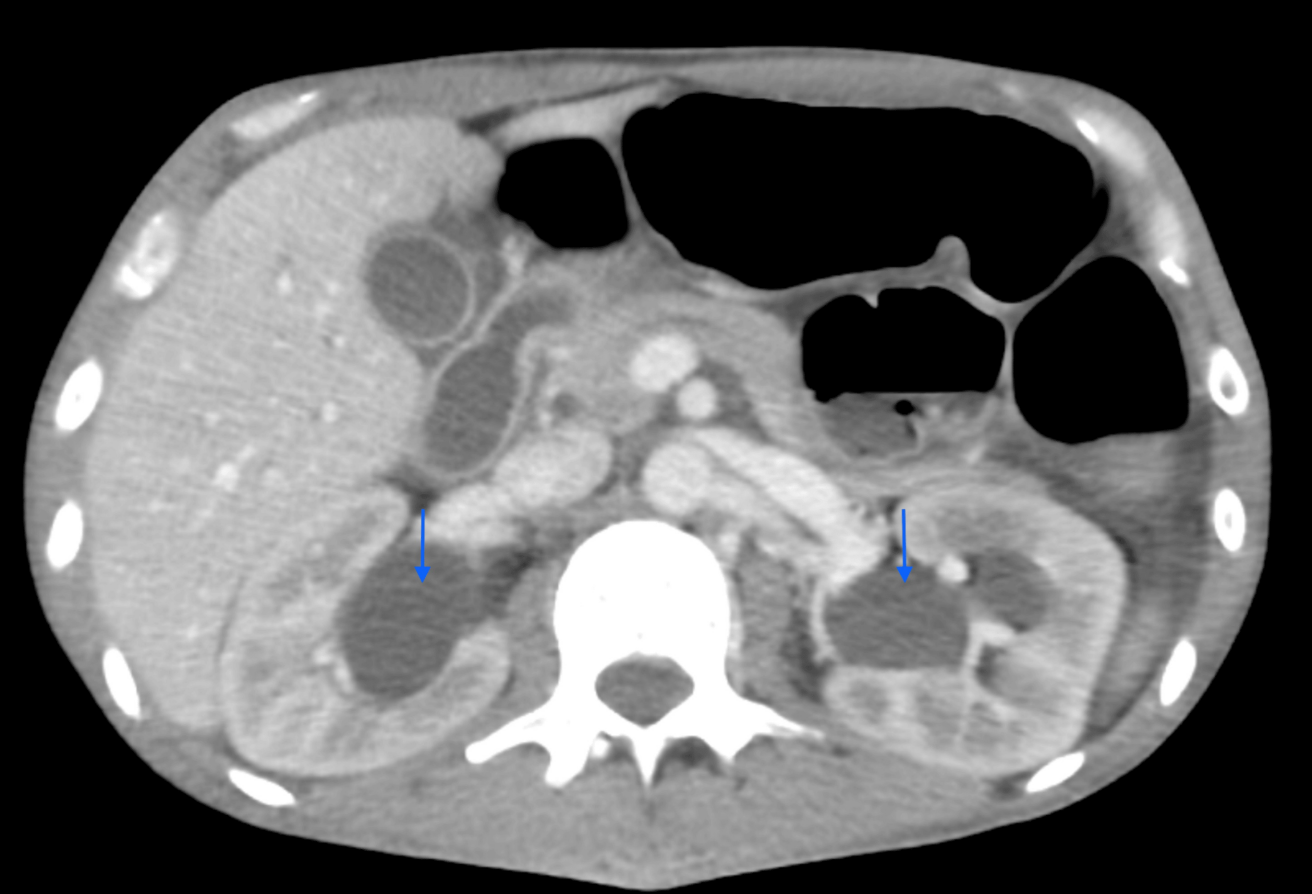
CT of abdomen and pelvis showing bilateral hydroureteronephrosis
